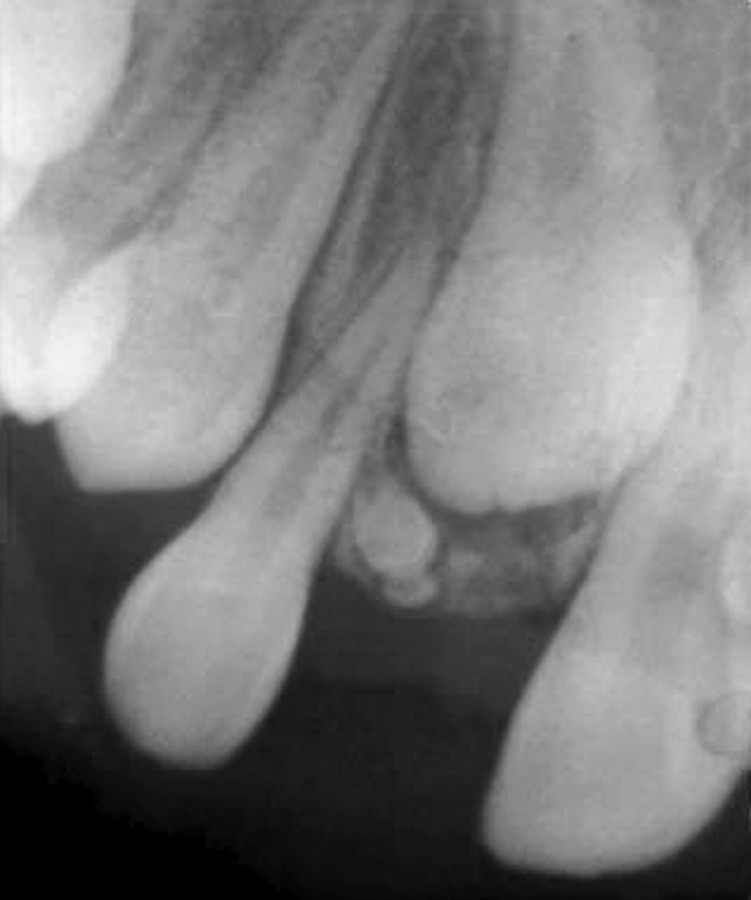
IOPA radiograph showing the presence of a
